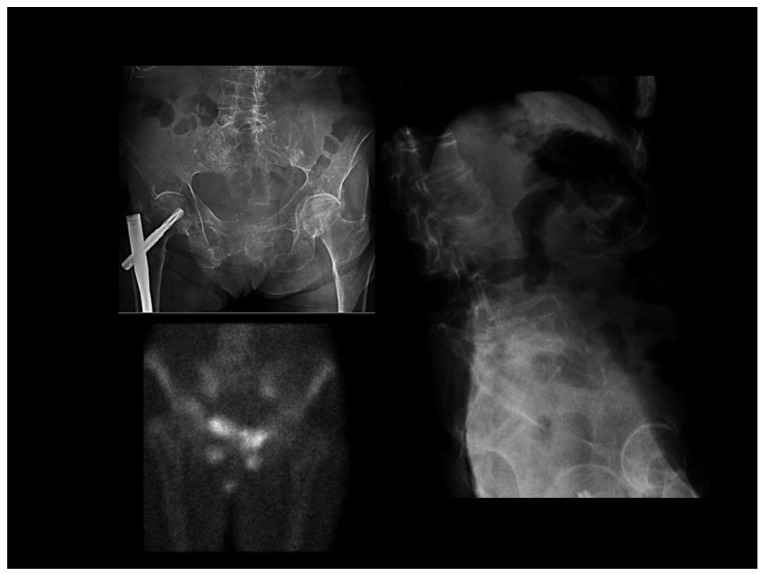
Multiple bone-insufficiency fractures in a patient with osteomalacia. Left upper part: fracture of the right hip (with surgical osteosynthesis) and pubic rami. Right side: multiple vertebral fractures. The lower-left picture shows a scintigraphic image consistent with fractures of the ilio and ischiopubic rami.
